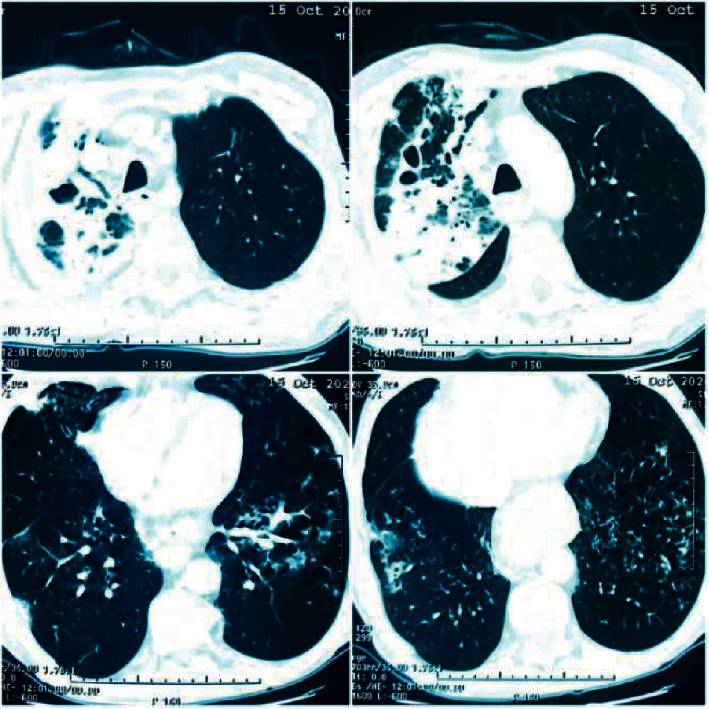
CT scan images showing bilateral micronodules and bronchial dilation in the right upper lobe with parenchymatous opacification. Endoluminal defects of the left pulmonary base suggesting a pulmonary embolism with signs of active TB infection.
